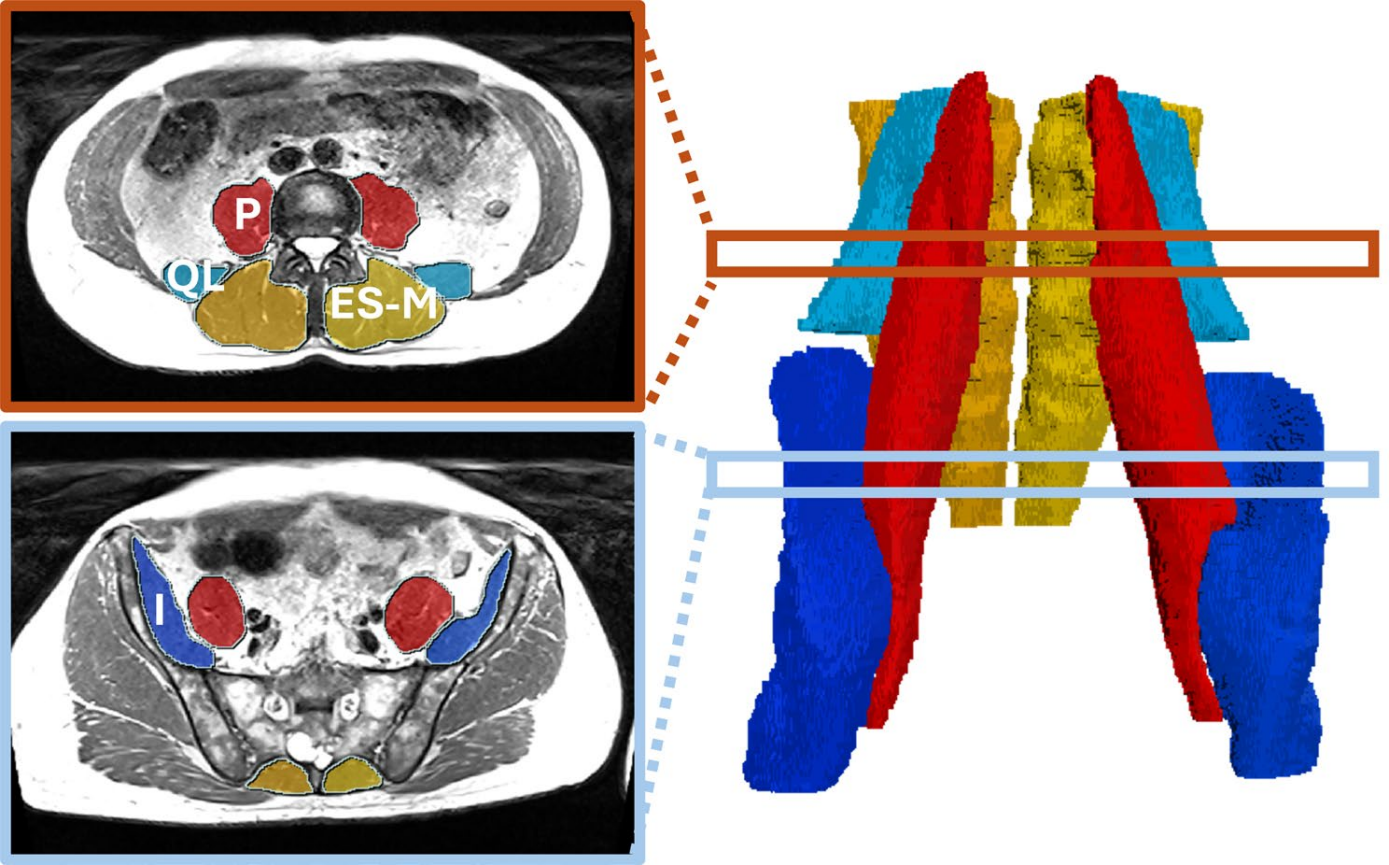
Example of a manual segmentation. On the right side, a 3D model of the manually segmented psoas (P), iliacus (I), quadratus lumborum (QL), erector spinae and multifidus muscles (ES + M). On the left, axial slices of the in-phase Dixon MRI with colour masks of the segmentations at two different levels of the spine
